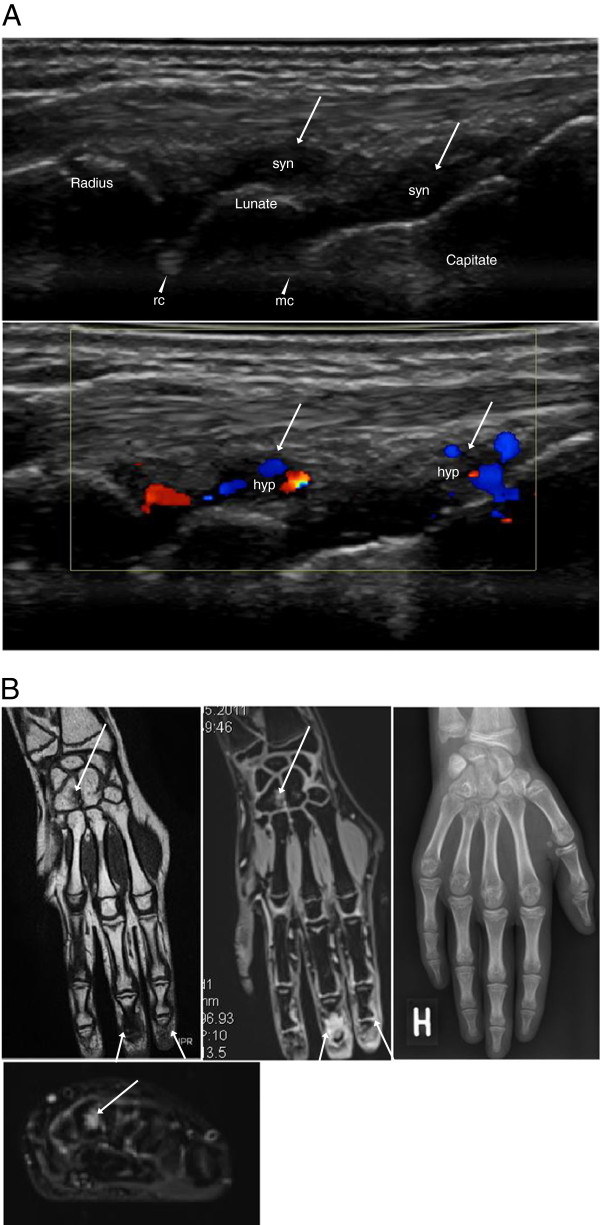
Imaging of the symptomatic wrist region of a 13-year-old girl with JIA. (A) Dorsal sagittal US scans of the radiocarpal (arrowhead, rc) and midcarpal (arrowhead, mc) joints showing synovial hypertrophy (syn, top image), with hyperemia (hyp) on color Doppler (bottom image), in the dorsal recesses (arrows). There are no visible erosions. (B) Overview of pathology in coronal MRIs of the hand and wrist. An erosion in the Hamate (arrow) and synovitis in the 2nd and 3rd DIP joints (arrows) are seen both in the coronal T1 SE image to the left, and in the coronal postcontrast 3D T1 GRE VIBE sequence in the middle. The axial STIR image (bottom image) reveals the bone marrow edema (arrow) surrounding the erosion of the Hamate. No pathology is displayed in the concurrent, posteroanterior X-ray (right image).
